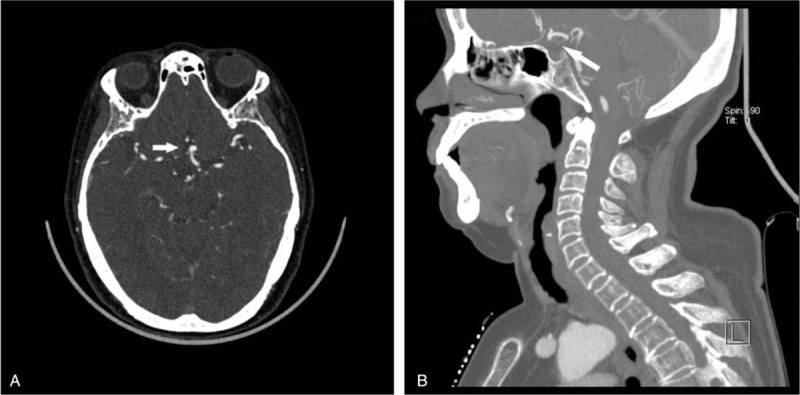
Axial (A) and sagittal (B) CTA images demonstrating an intracranial artery aneurysm of the anterior communicating artery (white arrow). This incidental finding was not initially reported on neck CTA.
